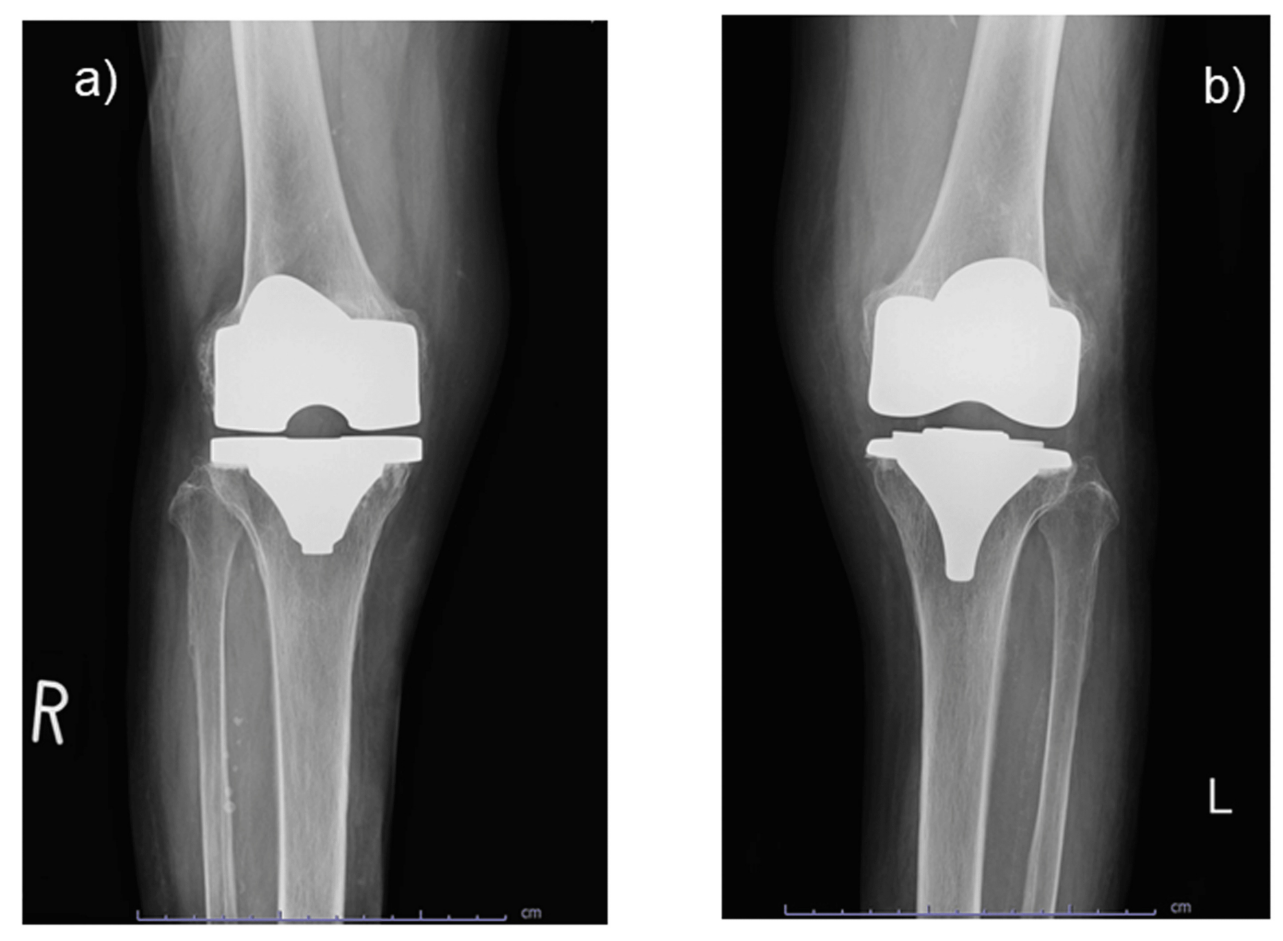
Radiography of both knees (a) Right knee. (b) Left knee. Knee radiography shows no fracture or prosthesis loosening.
